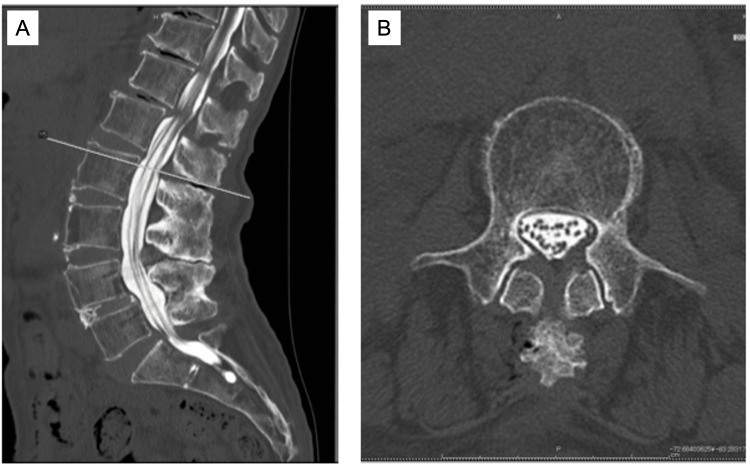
CT myelography images in Case 2. (A) Sagittal CT myelography performed immediately after contrast injection, showing no evidence of obstruction or hematoma. (B) Axial CT myelography showing preserved contrast flow without compression of the thecal sac CT: computed tomography
